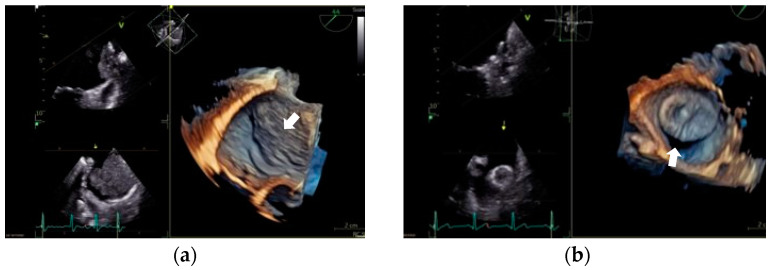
Primary postprocedural complications. (a) Device-related thrombus. Transesophageal echocardiogram. Midesophageal plane at 33°. It shows a large thrombus (46 × 35 mm) that covers the entire device and extends along the entire surface of the Marshall ligament. (b) Peri-device leak. Transesophageal echocardiogram. Midesophageal plane at 33°. It shows a significant gap between the pulmonary ridge and the device.
